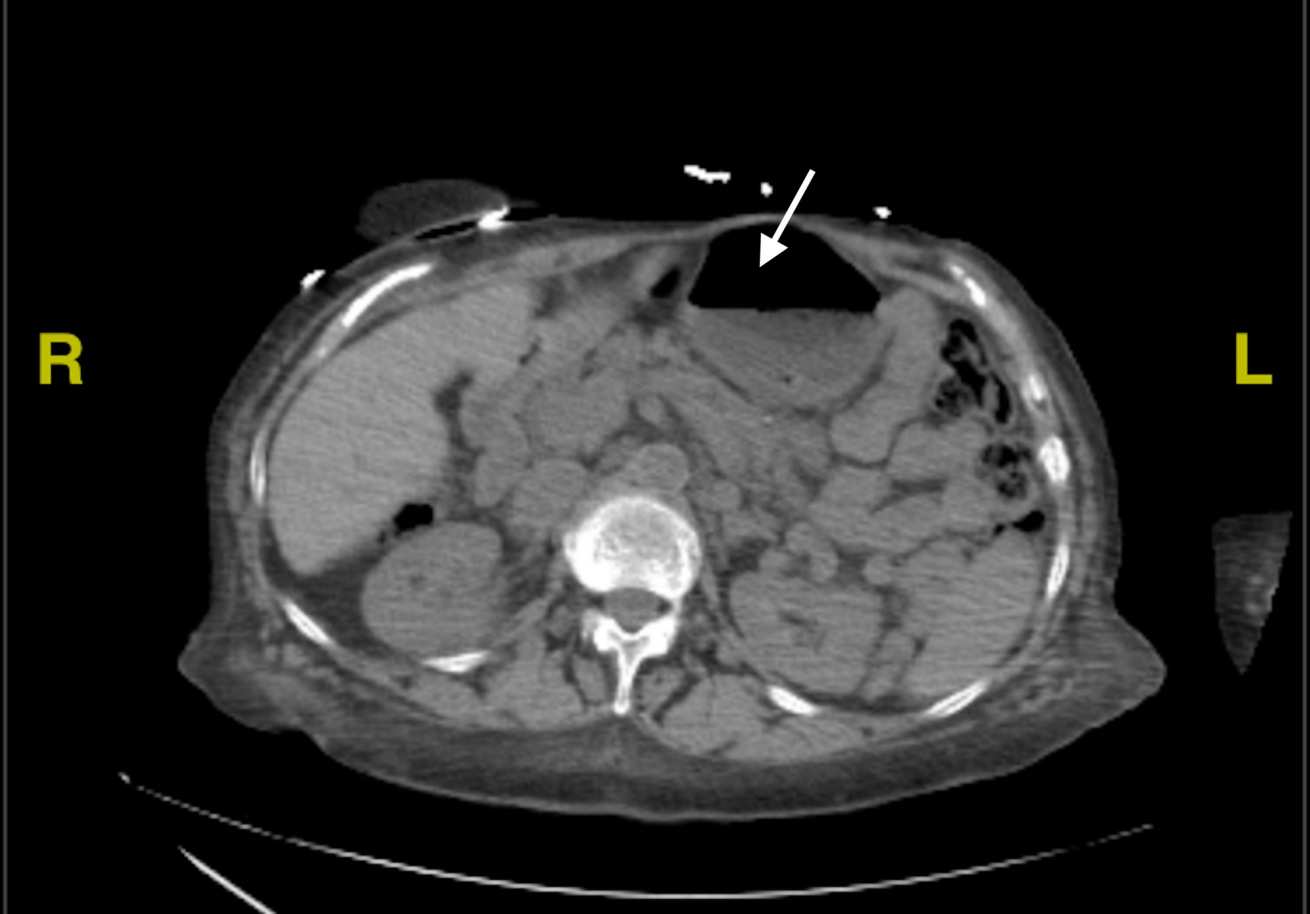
CT Abdomen and Pelvis showing gastric remnant with collection of blood Arrow shows Gastric remnant with collection of blood with in remnant
